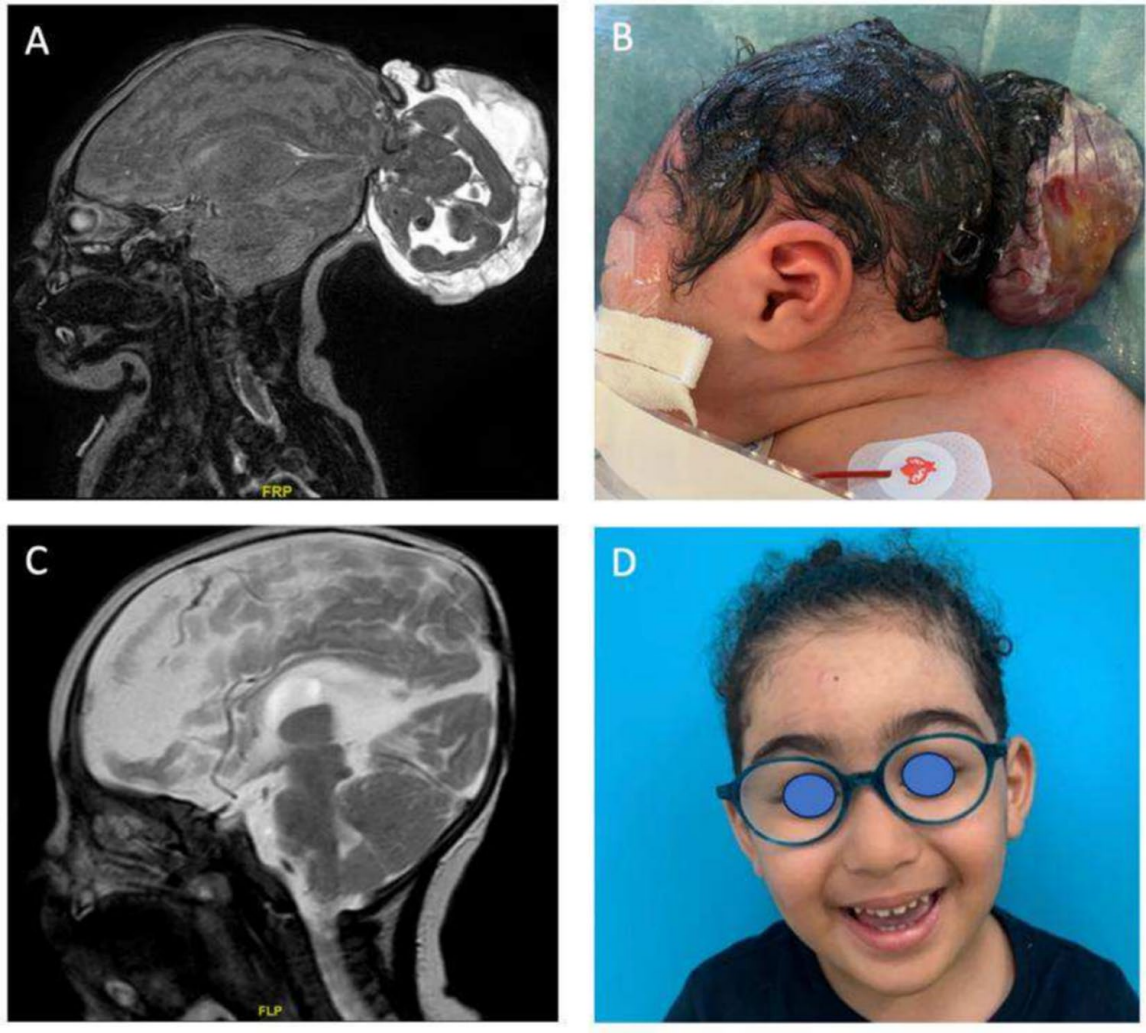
Occipital encephalocele, before and after surgical correction. A Postnatal sagittal T2-weighted MRI: we can appreciate the herniation of formed neural structure at occipital level; B pre-operative image of occipital encephalocele; C 5 years post-operative T2-weighted sagittal MRI showing the complete correction of the encephalocele; and D patient aspect at the follow-up
